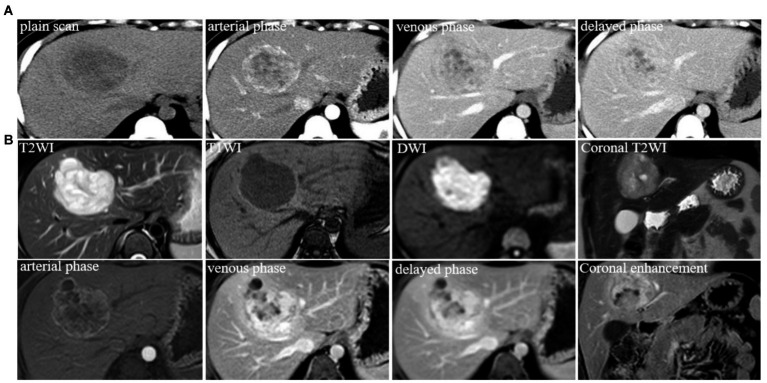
(A) Typical level and imaging findings of abdominal CT images for the lesion in the S4 segment of the liver; (B) abdominal MR typical images for the lesion in the S4 segment of the liver.
